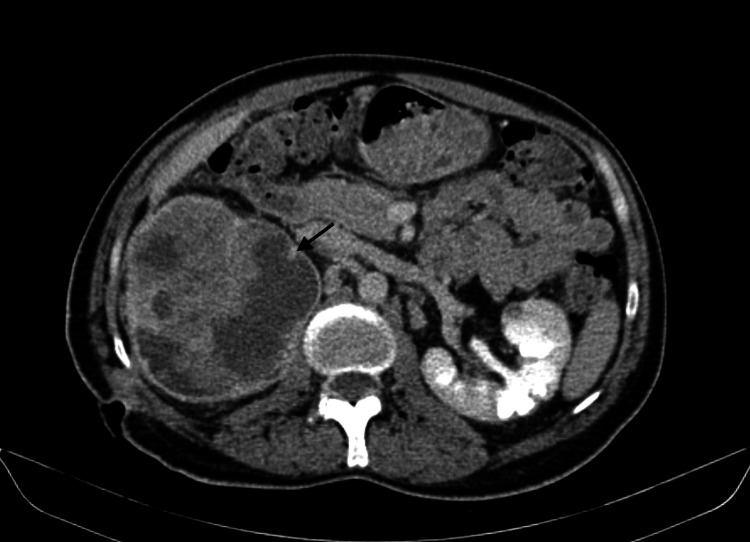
An intravenous contrast-enhanced abdominal CT section showing a giant cystic mass with solid components and heterogeneous contrast uptake with an arrow. The edges of the mass are well-defined, it has a capsule-like contrast enhancement, and the nephrocutaneous fistula is seen.
